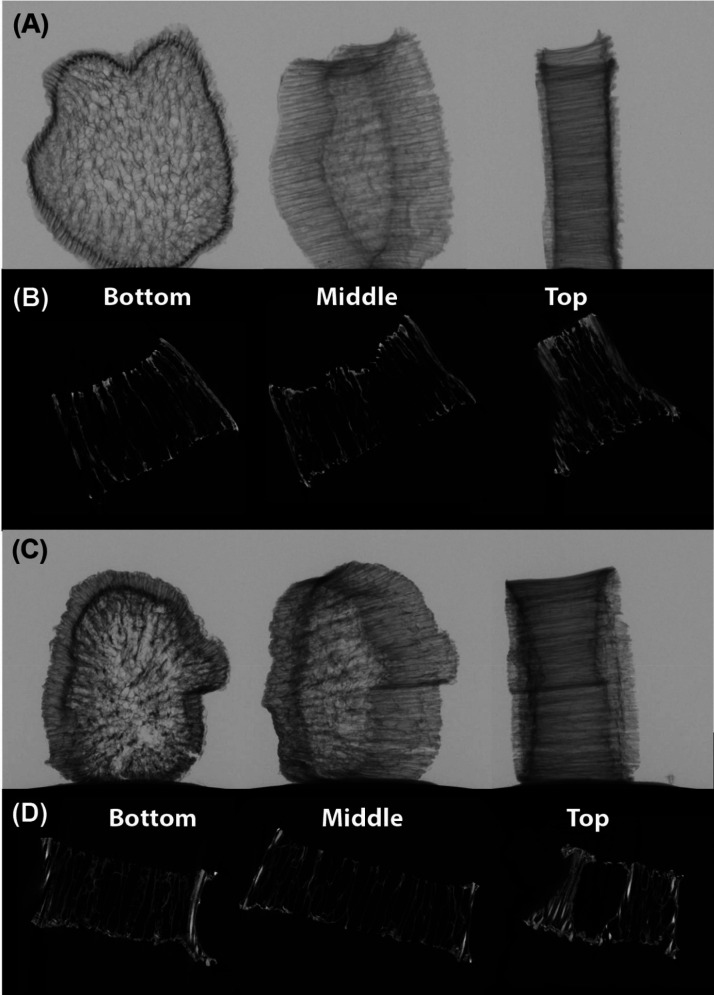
X-ray transmission image of (A) SD/Si and its
